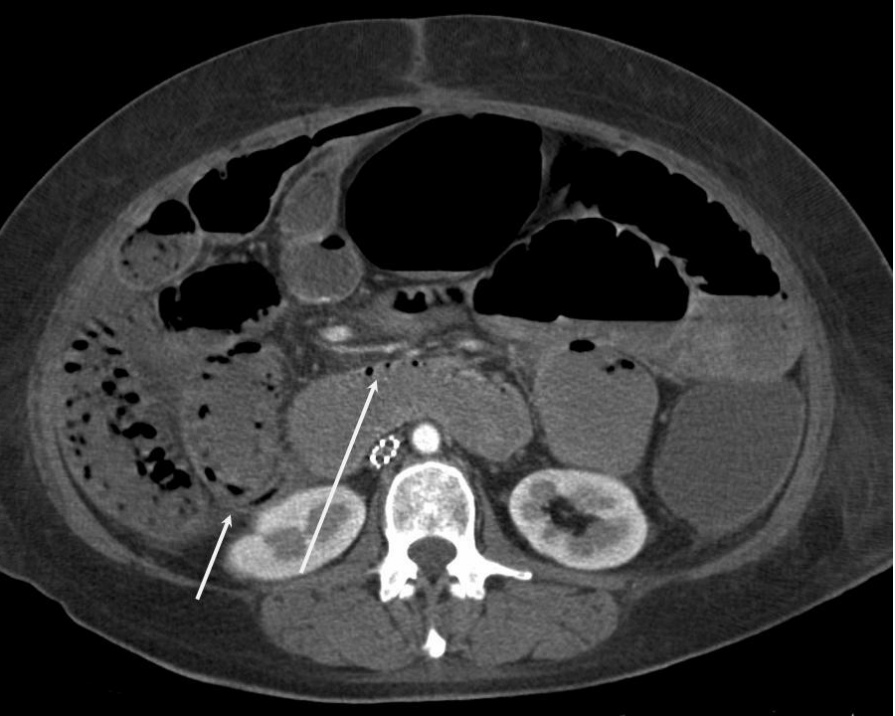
Computed tomography demonstrating pneumatosis intestinalis within the walls of the small and large bowel (arrows).
